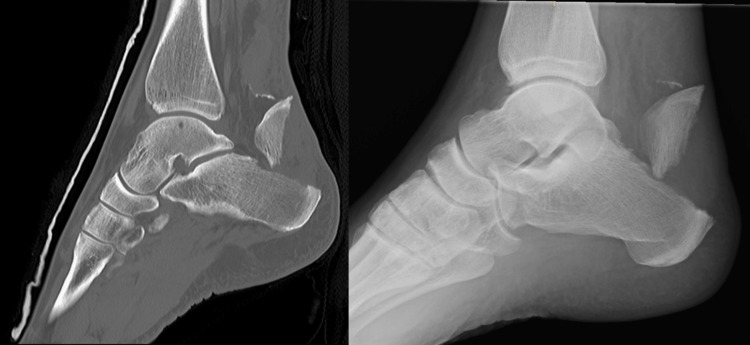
A sagittal CT (right) and lateral X-ray showing the fracture fragment and further small avulsion fragment CT: computed tomography
